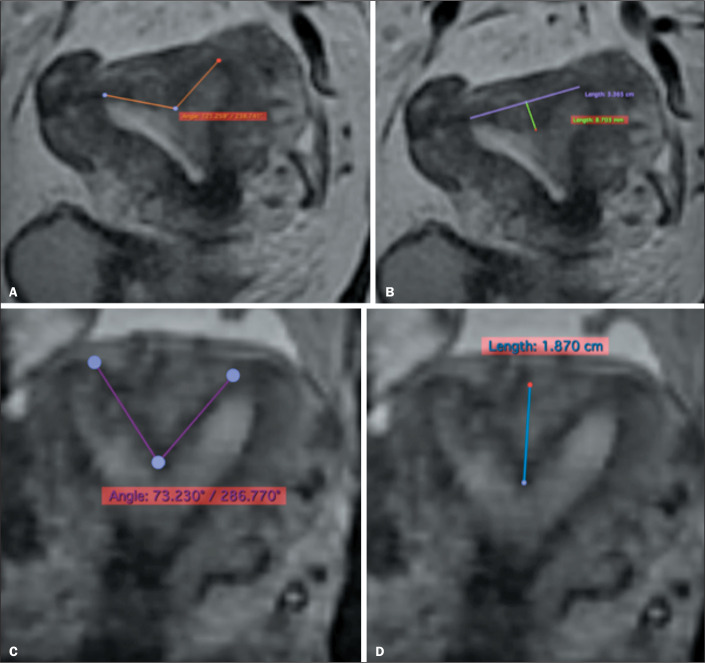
T2-weighted, volumetric, turbo spin-echo MRI scans showing how to
